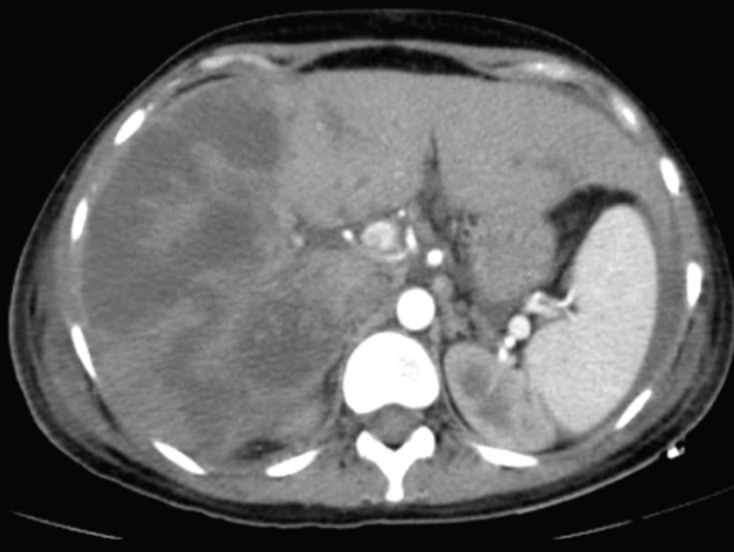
contrast-enhanced CT of the abdomen showed the damaged areas were persisted on the 15^th^ days after admission
